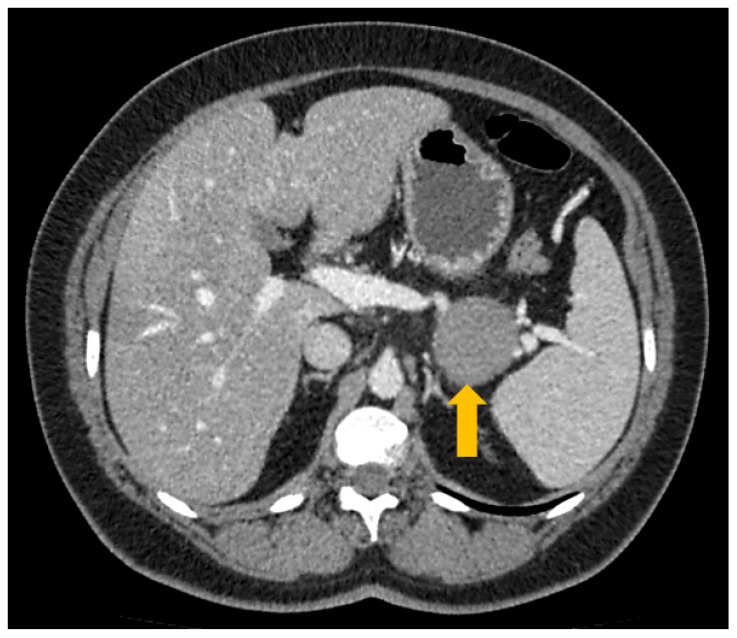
CT scan showing the presence of a roundish mass (4.5 cm, yellow arrow) between the stomach and the spleen.
